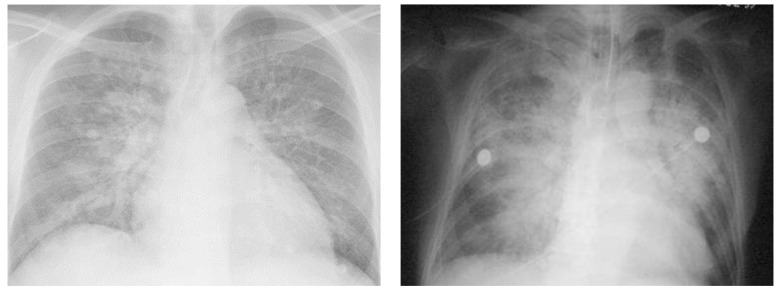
Chest Radiographs representing Cardiogenic and Noncardiogenic Pulmonary Edema. Left X-ray shows an anteroposterior chest Rx with acute anterior myocardial infarction and acute cardiogenic pulmonary edema. Signs of cardiogenic pulmonary edema are peri broncho vascular spaces and the prominent septal lines (Kerleyșs B lines). Right X-ray shows an anteroposterior chest Rx with noncardiogenic pulmonary edema, with blood culture positive for Streptococcus pneumoniae, causing pneumonia complicated by septic shock and acute respiratory distress syndrome. Diffuse alveolar infiltrates appear patchy and bilateral with air bronchograms, findings that are charac-teristics of, but not specific to, noncardiogenic edema and acute lung injury.
